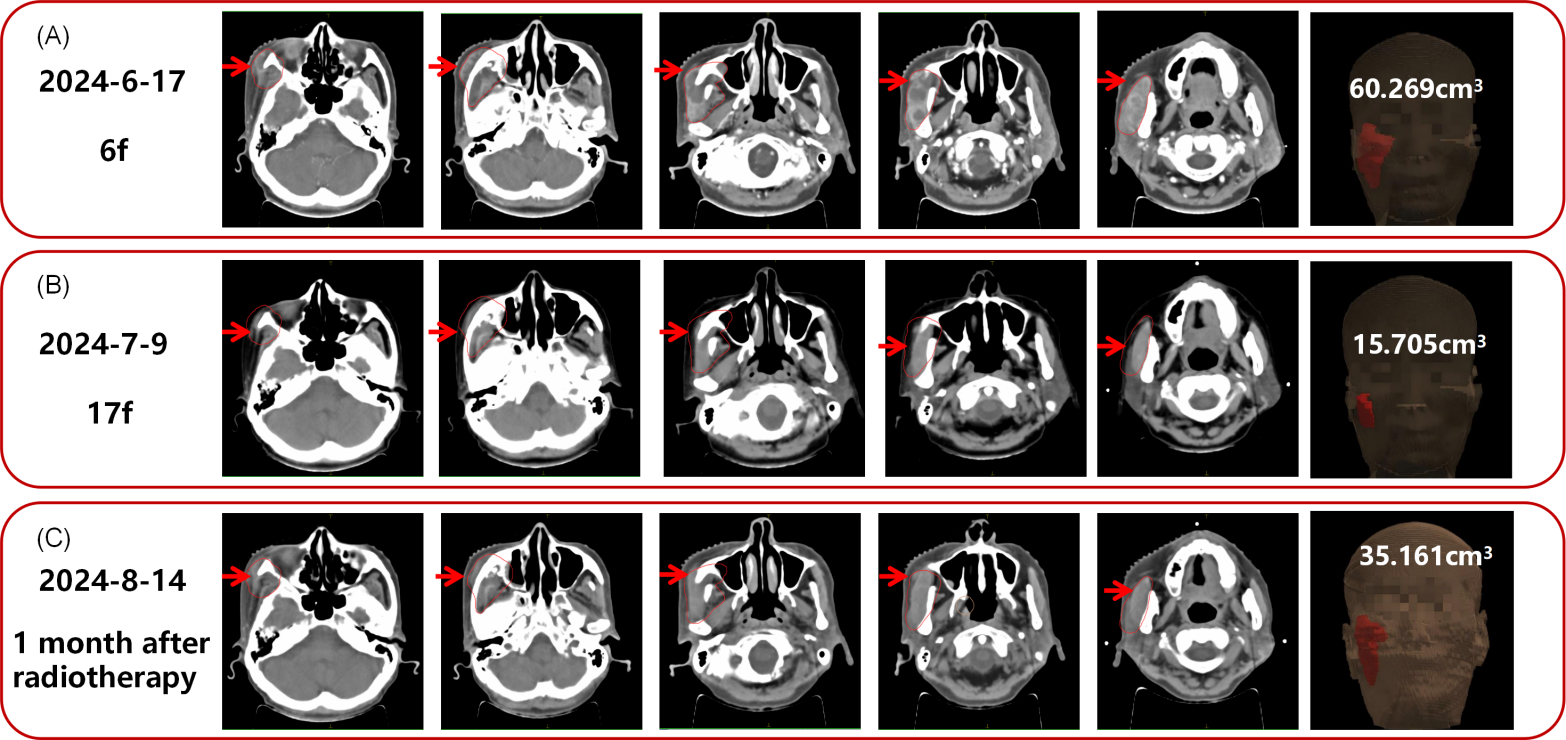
CT images of the patient’s maxillofacial region. (A) At the 6th radiotherapy fraction. (B) At the 17th radiotherapy fraction. (C) One month after the completion of radiotherapy.The red contour indicates the tumor delineation on June 17, 2024, serving as a baseline for comparison with subsequent tumor changes. The red arrow points to the tumor location, and the tumor volume is labeled on the last image of each time point.
